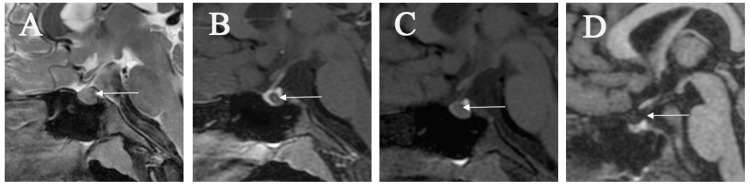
(A) MRI-T2 sagittal view: preoperative MRI-T2 weighted sagittal view shows a well-shaped pituitary gland with nodular hypointense areas within the pituitary (white arrow). (B) MRI-T1 enhanced sagittal view: preoperative pituitary MRI enhanced examination reveals a nodular non-enhancing area between the anterior and posterior lobes of the pituitary (white arrow), measuring approximately 0.6 × 0.6 × 1.3 cm in size. (C) MRI-T1 sagittal view: preoperative MR-T1 weighted image displays a high-signal area in the posterior lobe of the pituitary (thick white arrow), with a T1 hypointense area anterior to the posterior lobe (white arrow). (D) Postoperative pituitary MRI-T1WI sagittal view: shows the disappearance of the previous cystic area within the pituitary, significant reduction in pituitary size, and good imaging of the high-signal area in the posterior lobe of the pituitary (white arrow).
